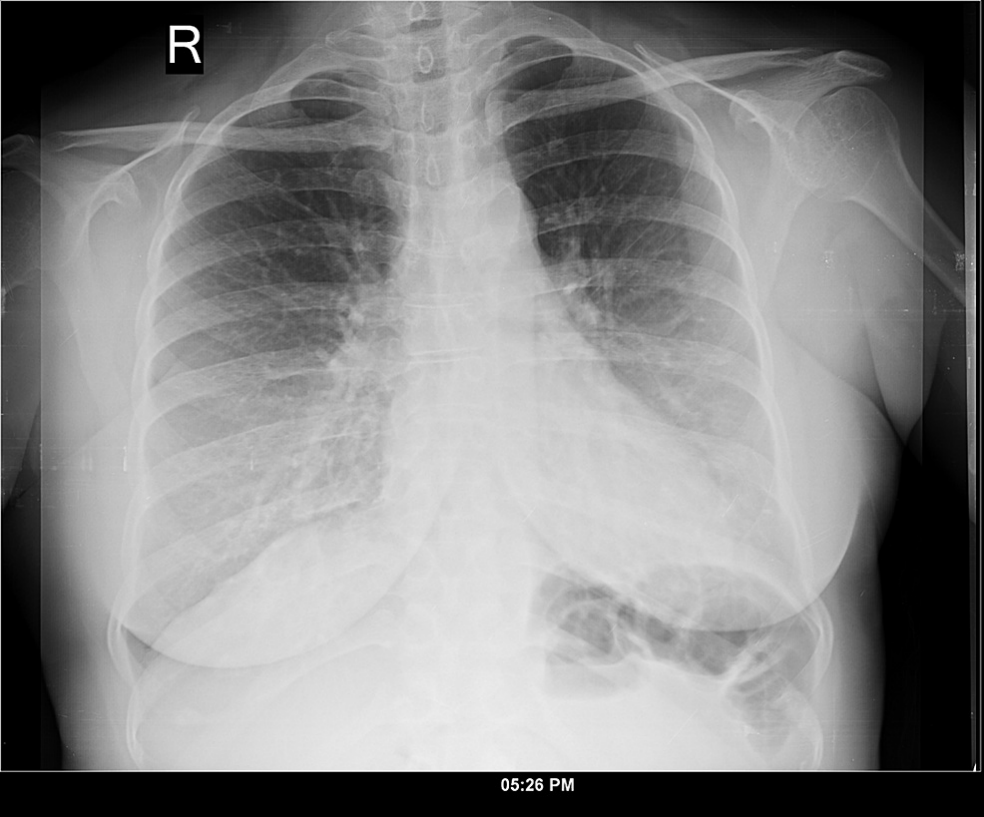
Chest X-ray posteroanterior view: taken three years after the surgery No pleural effusion is seen.
